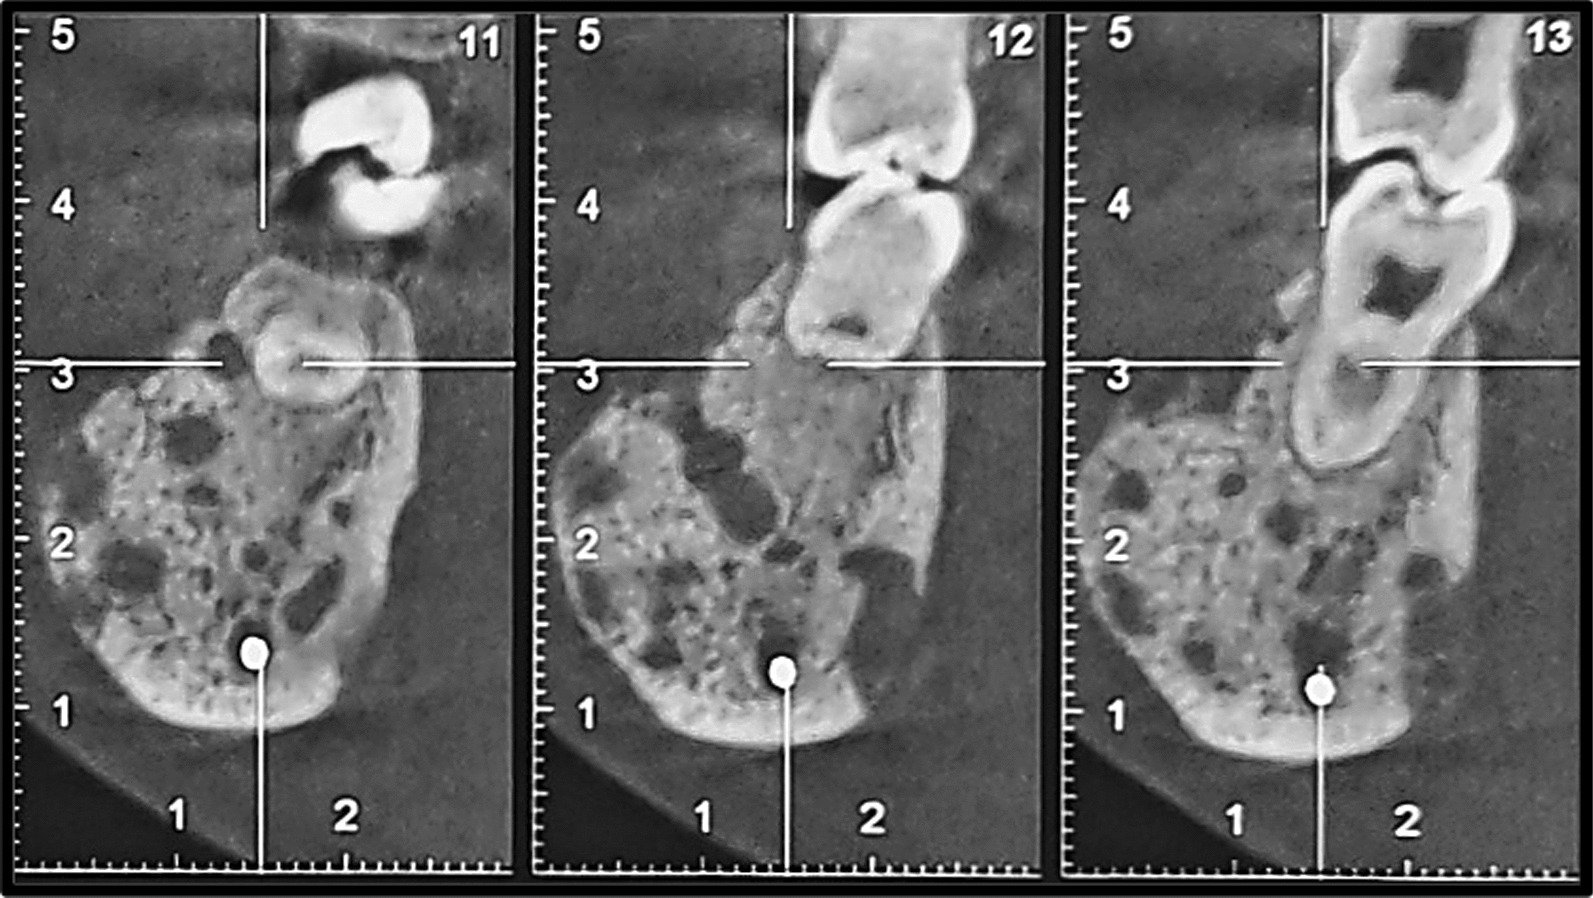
Cone bean computed tomography image (cross-sections). Erosion of alveolar crest, expansion, thinning and loss of continuity of both buccal and lingual cortices are evident
